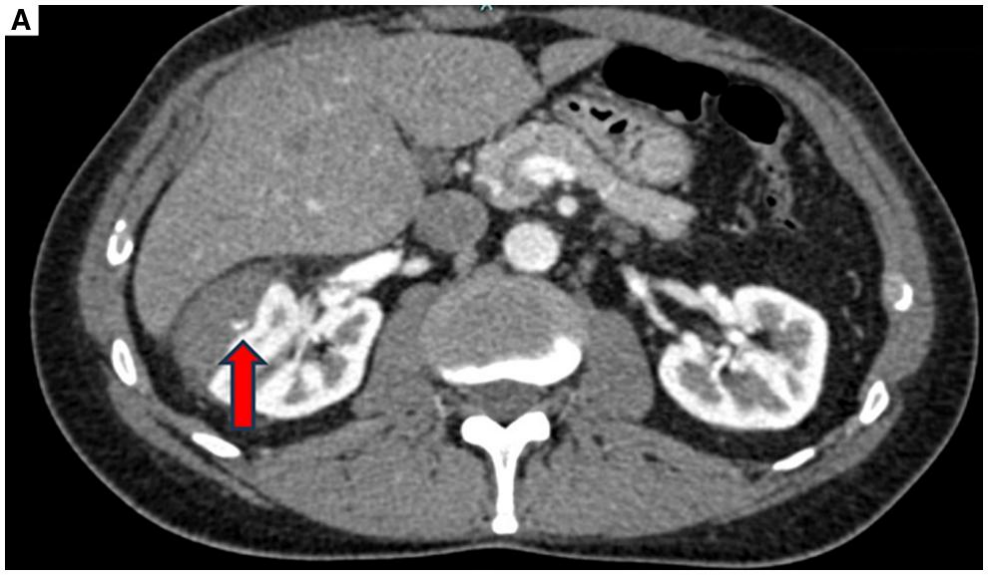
Active bleeding. (A) Axial CT image in the arterial phase demonstrating a right renal hematoma with active bleeding (arrow). Abbreviation: CT = computed tomography.
